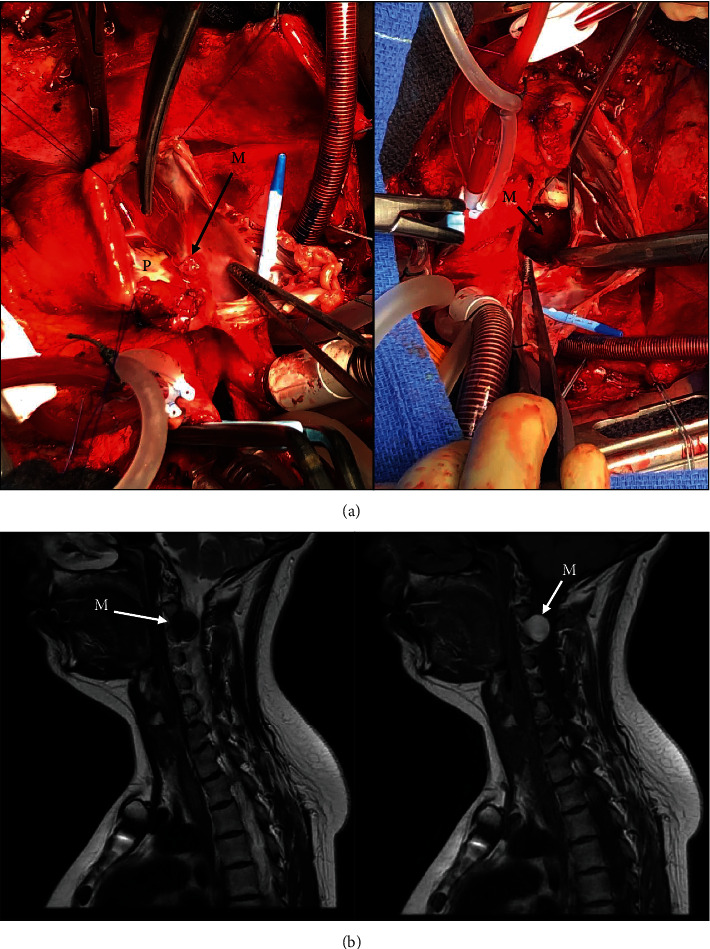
(a) Operative views. Left part: after right atriotomy and before septotomy: a small 7 × 6 mm mass (M) enshrined below the insertion of the previous pericardial patch (P) used to reconstruct the interatrial septum 13 years earlier. Right part: after septotomy, a gelatinous and mobile mass (M) is easily visualized into the left atrium. (b) Cervical MRI performed 11 months after the resection of the myxoma depicts a 20 × 18 mm cervical intradural spherical mass (M) at the C2-3 level with signs of spinal cord compression. Left part: T2-weighted sagittal image. Right part: T1-weighted sagittal image.
